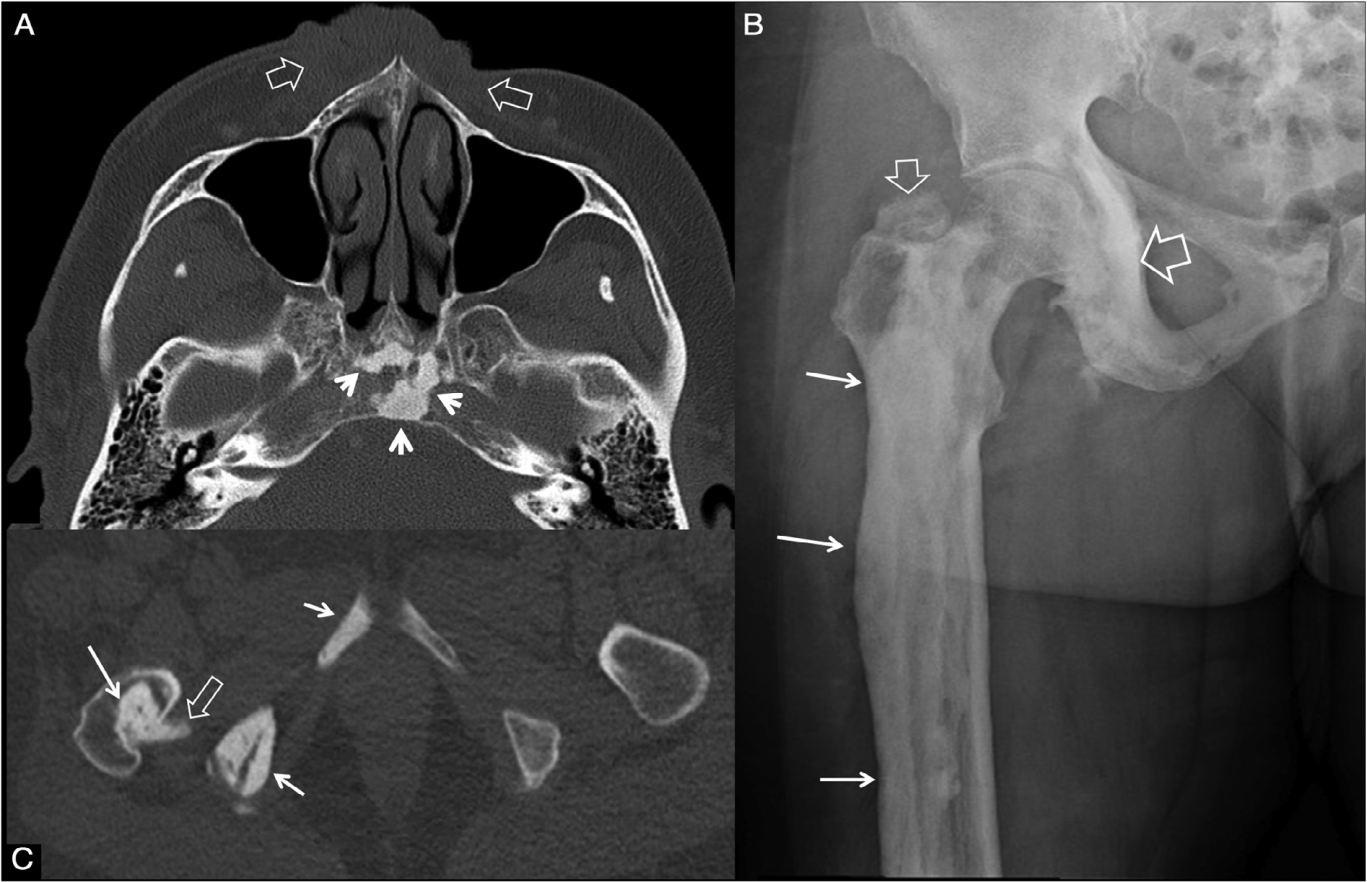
Value of CT in melorheostosis. A 60‐year‐old female patient (Melo‐13) with a follow‐up of 10 years after initial diagnosis. (*A*) Axial CT scan showing endosteal hyperostosis in the clivus (arrows) and subcutanous soft tissue lesions in the face on both sides of midline (open arrows). (*B*) Anteroposterior plain X‐ray of the right femur showing an extensive endosteal hyperostosis lesion in the proximal metadiaphysis of the femoral bone (arrows) and the ischiopubic bone (open arrow). A soft tissue ossification is shown above the greater trochanter (small open arrow). (*C*) Axial CT, bone window, in the pelvis, shows endosteal hyperostosis in the femoral (arrow) and ischiopubic bone (small arrows). Parosteal bone is extending into the ischiofemoral space, located into the quadratus femoris muscle (open arrow).
